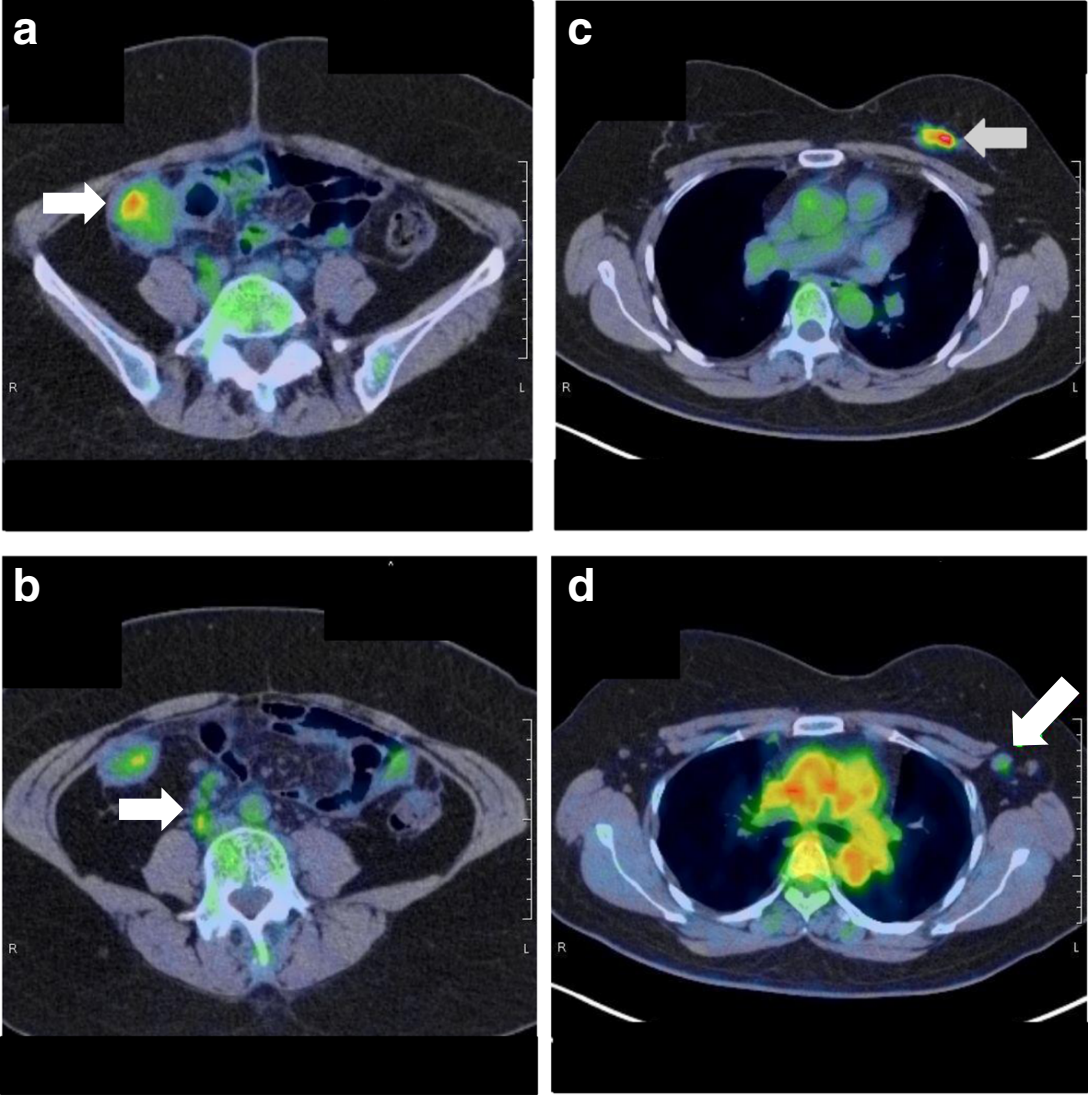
Positron emission tomography-computed tomography (PET-CT). a A whole PET-CT image shows fluorodeoxyglucose (FDG) uptake in the ascending colon. The maximum standardized uptake value (SUVmax) is 5.0. b A whole PET-CT image shows FDG uptake in the mesentery. The SUVmax is 2.8. c A whole PET-CT image shows FDG uptake in the left breast. The SUVmax is 6.4. d A whole PET-CT image shows FDG uptake in the left axillary region. The SUVmax is 2.1
